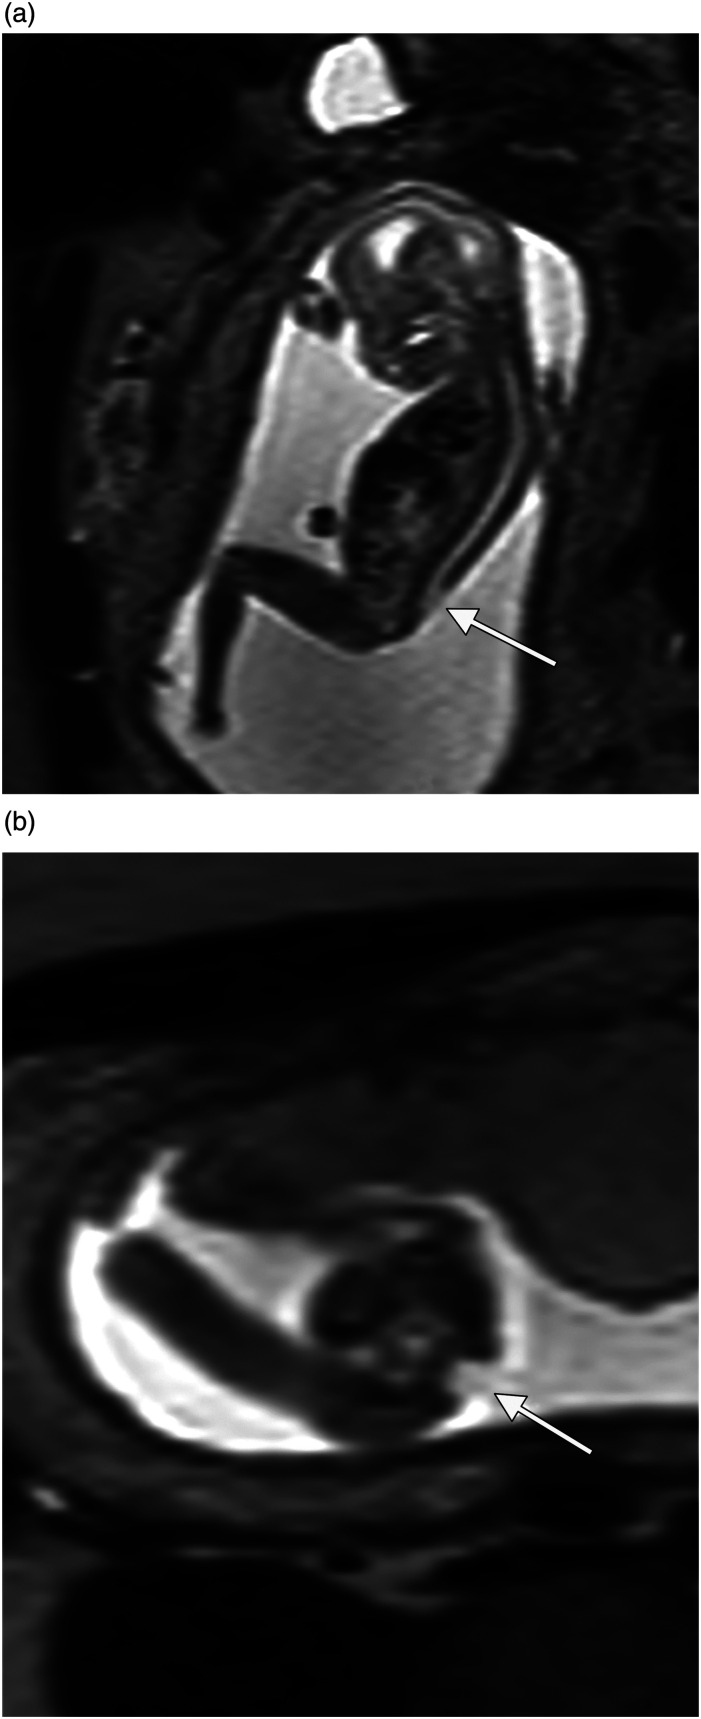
(a) and (b). Case no 2. Ultrasound examination revealed lemon-shaped head. Sagittal (a) and axial (b) MR images show low lying cerebellum but also lumbosacral spinal closure defect (arrows) as in myelomeningocele.
